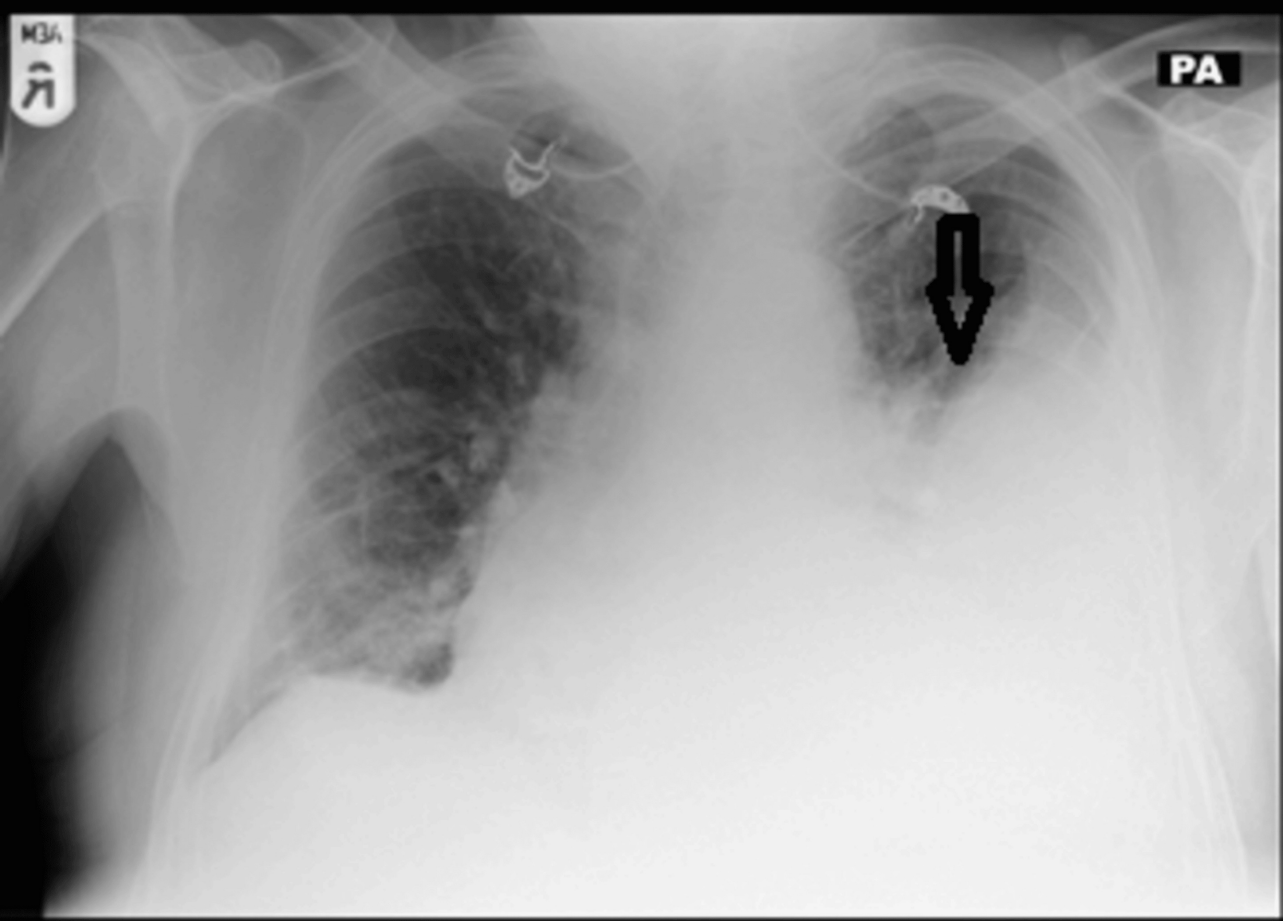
Admission posteroanterior view chest X-ray showing left pleural effusion
